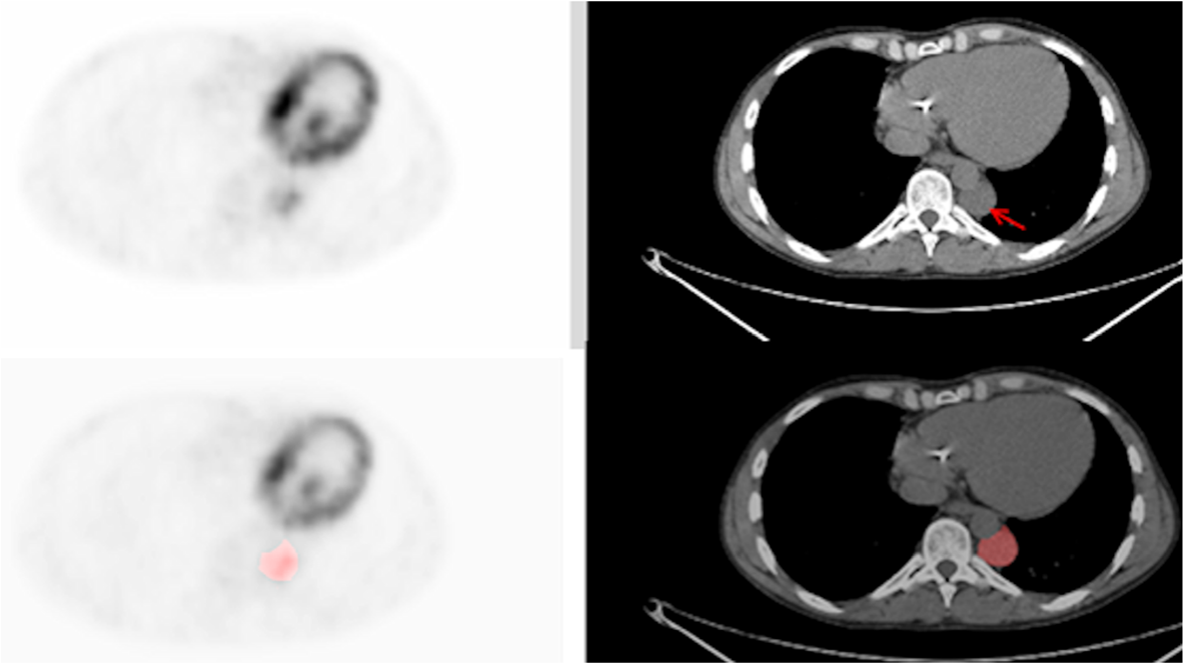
Axial ^18^F-FDG PET and CT scans. The tumour is indicated by the *arrow* (*above*). A region of interest is shown on the same PET and CT slice of the tumour (*below*)
